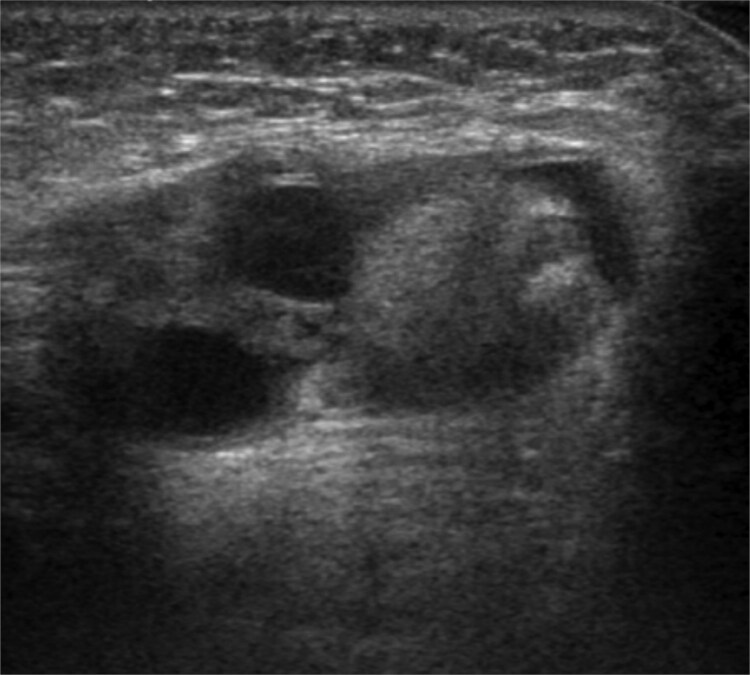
Ultrasonography revealed a 12 mm-sized circular highly echogenic mass and 7- and 8-mm-sized cystic masses within the hernia sac. Ipsilateral ovarian herniation was suspected.
